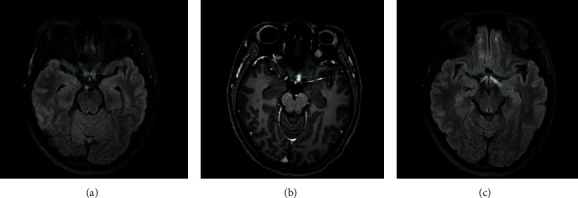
(a): FLAIR Axial image showing thickening and increased signal intensity in the optic chiasm. (b): T-1 weighted post Gadolinium contrast Axial image showing enhancement in the left side of the optic chiasm. (c): FLAIR Axial image showing increased signal intensity in the bilateral optic tracts. (Left > right).
